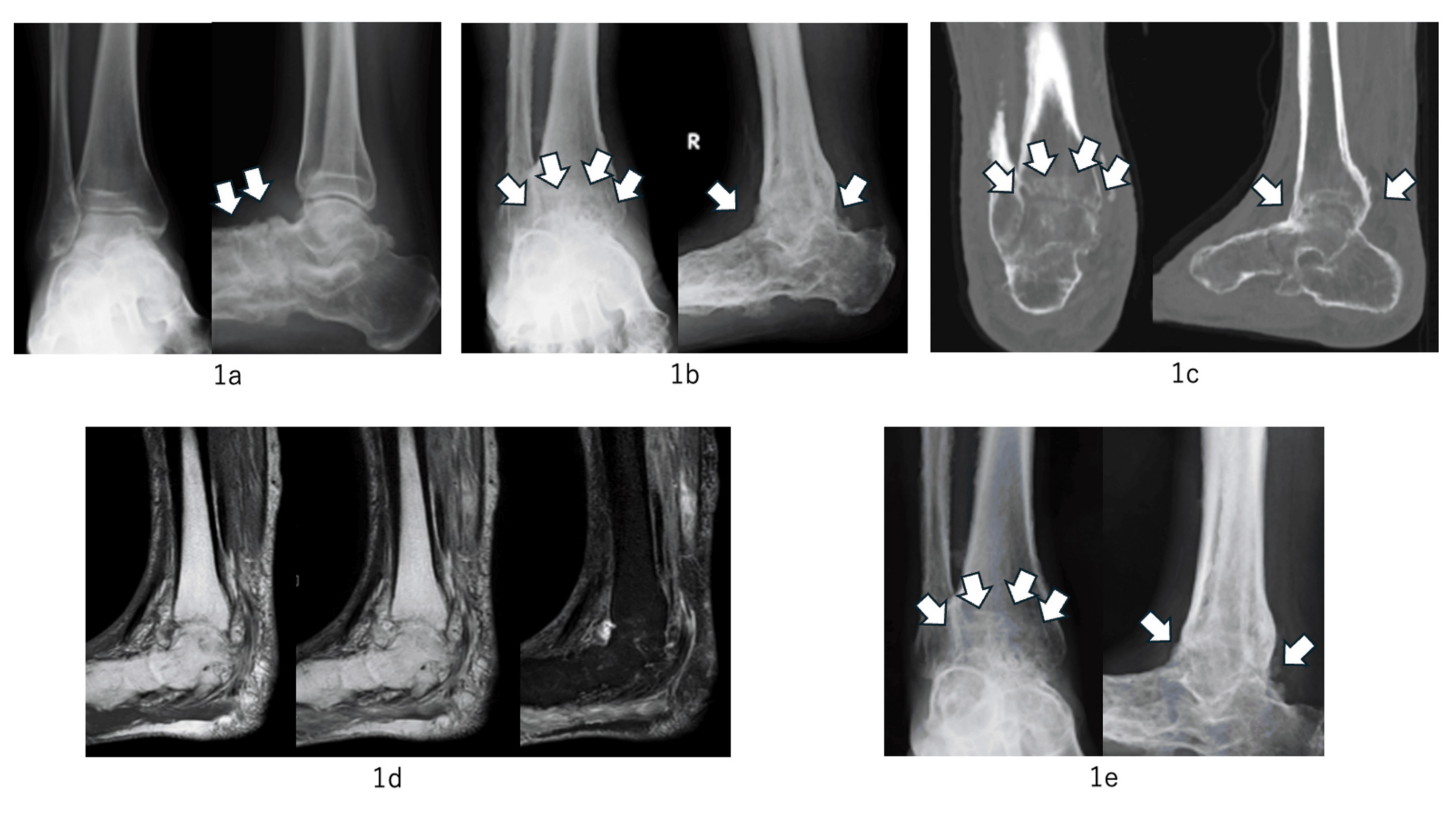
Image findings X-rays of the right ankle 15 years ago. There was no destruction of the ankle joint 15 years ago, but bone erosion and joint space narrowing of the midfoot were seen (1a, point out joint space narrowing of the midfoot, white arrow). X-rays and computed tomography (CT) of the right ankle when he referral to our hospital. bone and joint destruction were observed with severe ankle ankylosis (1b; X-ray, point out ankle ankylosis, white arrow. 1c; CT, left; sagittal view, right; coronal view, point out ankle ankylosis, white arrow.). Magnetic resonance imaging (MRI) of the right ankle upon referral to our hospital. MRI revealed no abscesses and no signal changes which were indicative of infection. (1d, left; T1WI sagittal, center; T2WI sagittal, right; DIWI sagittal) Upon the last examination, the joint destruction and ankylosis of the ankle slightly progressed compared to the initial evaluation at our hospital (1e, point out ankle ankylosis, white arrow.).
